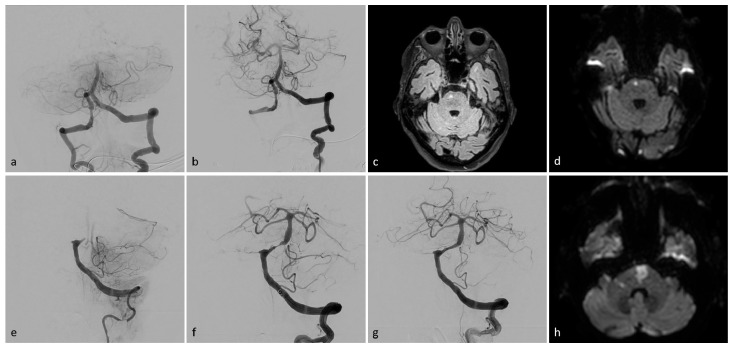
Exemplary patient imaging. Intraprocedural angiographic images (a,b,e–g), fluid-attenuated inversion recovery (FLAIR), magnetic resonance imaging (MRI, (c)), and diffusion-weighted images (DWI, (d,h)). Patient I (a–d): A 73-year-old man presented with left-sided weakness and vertigo, followed by a coma. Initial angiography revealed occlusion of the basilar head (a). Successful TICI 3 recanalization was achieved after a single aspiration thrombectomy (b). MR-imaging showed a small paramedian pontine infarct on the right side (c,d). The patient was discharged with mild left-sided hemiparesis (mRS 2). Patient II (e–h): A 78-year-old woman presented with acute-onset tetraplegia. Initial angiography (e) showed proximal occlusion of the basilar artery. Post-thrombectomy, high-grade stenosis was observed in the proximal third of the basilar artery (f). After percutaneous transluminal angioplasty (PTA) and stenting, the stenosis resolved, with a final result of TICI 3 (g). DW imaging revealed an infarct in the pons and a small infarct in the right cerebellar peduncle (h). The patient was discharged with persistent tetraparesis, unable to walk without assistance (mRS 4).
